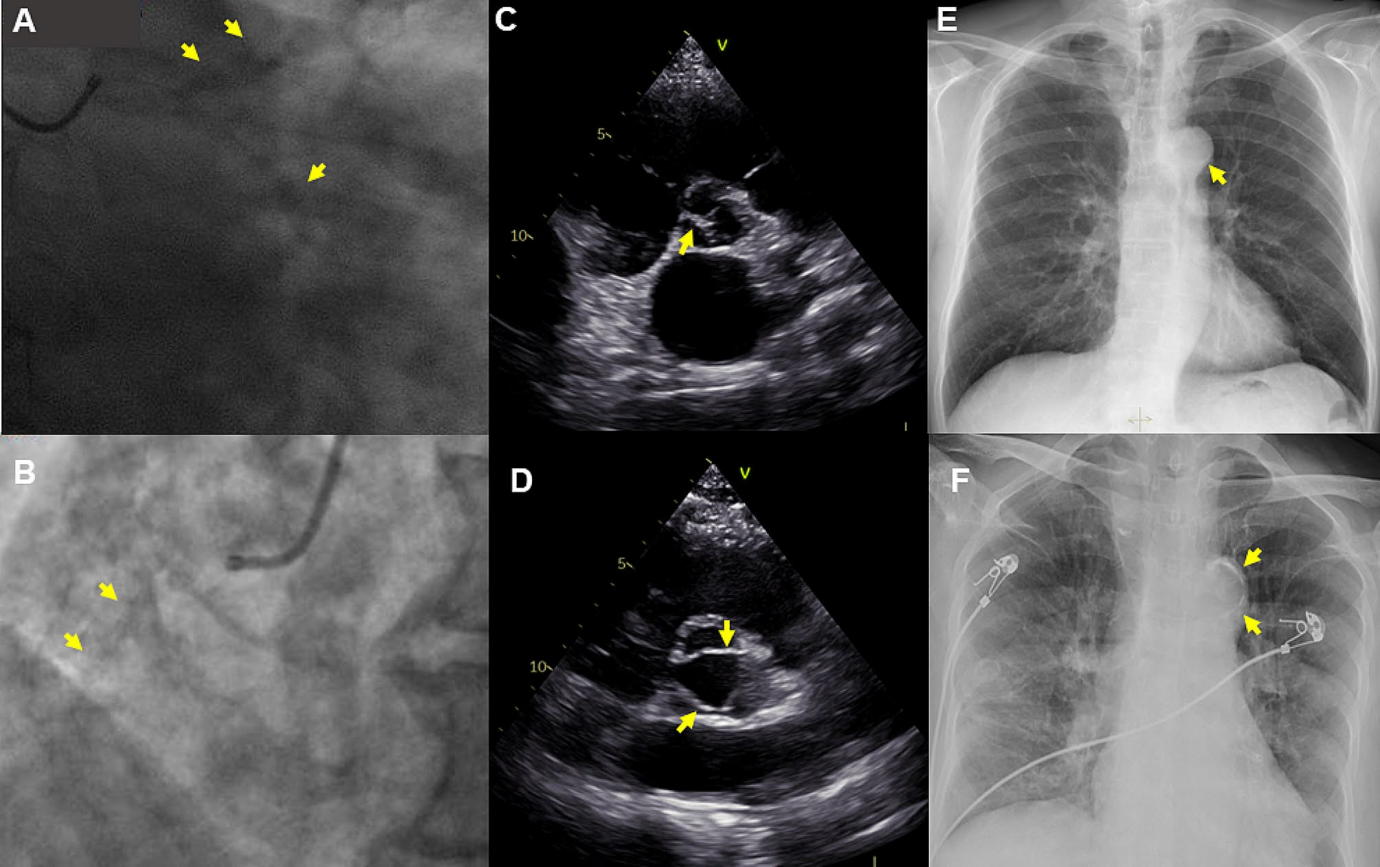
(A and B) Coronary artery calcification (CAC) in coronary angiography. (C and D) Aortic valve calcification (AVC) in echocardiography. (E and F) Thoracic aortic calcification (TAC) in chest X-ray
